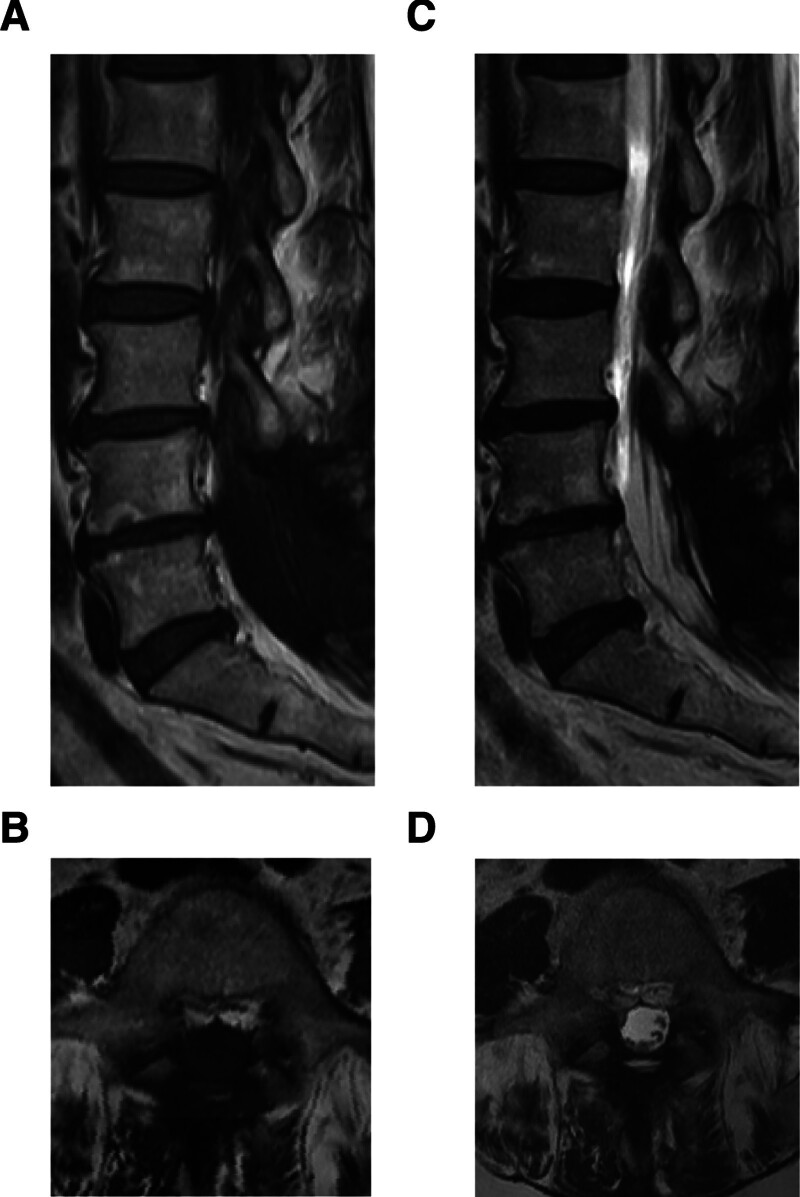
Postoperative imaging findings of the clear cell meningioma (CCM). (A and B) One year postoperatively, T1-weighted MRI in the sagittal (A) and axial (B) sections. (C and D) Postoperative T2-weighted MRI in the sagittal (C) and axial (D) sections.
